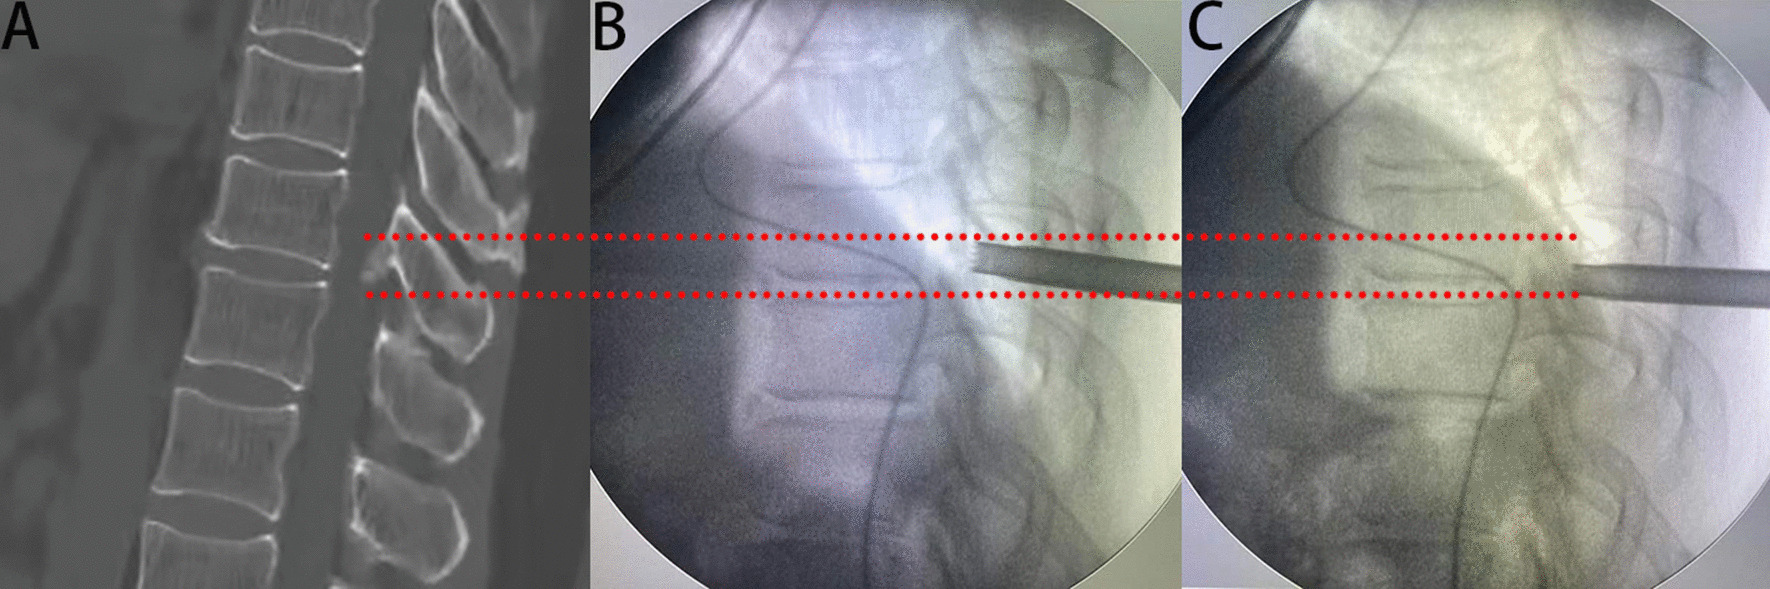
A Preoperative CT (sagittal). B Mark the cranial boundary of ossification on the surface of the lamina with the trephine. C Mark the caudal boundary of ossification on the surface of the lamina with the trephine. Dotted line; Roughly delimit the removal range of the lamina
